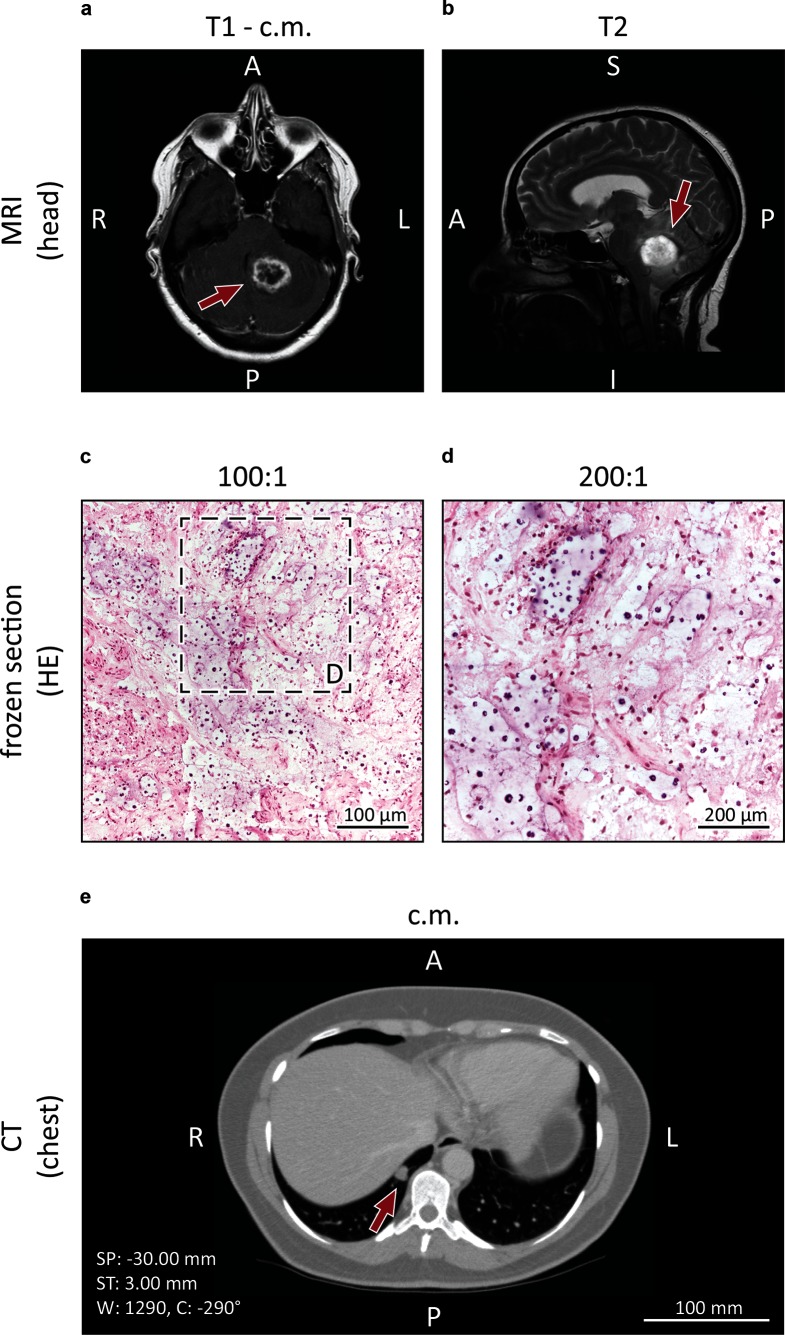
Preoperative and intraoperative diagnostic investigations. Cranial magnetic resonance imaging (a and b) showing a circumscribed mass in the left cerebellum hemisphere (arrows). Further investigations included a chest computed tomography (e) with presentation of a subpleural nodule in the right lower lobe (arrow). An intraoperative frozen section (c and d) revealed paucicellular glial tissue with several infiltrating immune cells.
